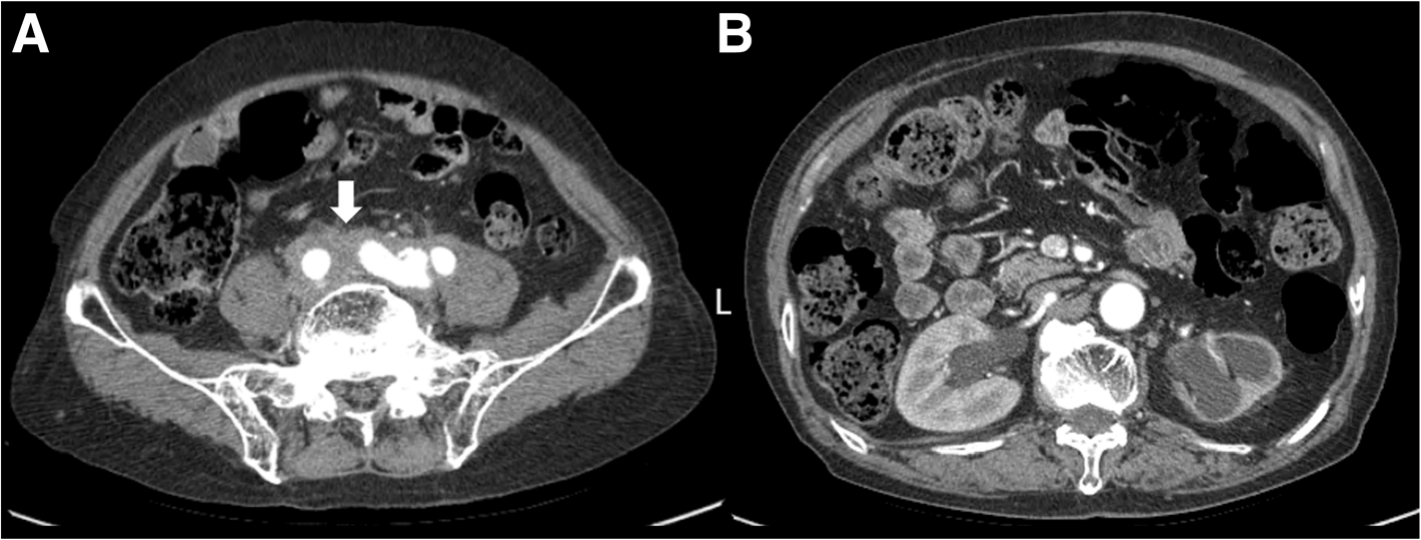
Abdominal computed tomography (CT). a The retroperitoneal mass surrounding the vessles ranging from the aortic bifurcation to presacral area (arrow). b Both hydronephrosis and atrophied left kidney
